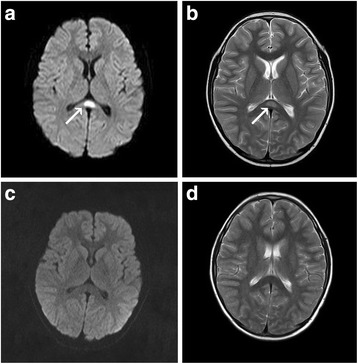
MRI findings of case 1. Diffusion-weighted image (a) and T2-weighted image (b) on the day of admission showed a focal high-signal lesion in the splenium of the corpus callosum (arrow). On the seventh day after admission, the splenial lesion had completely disappeared on diffusion-weighted image (c) and T2-weighted image (d)
